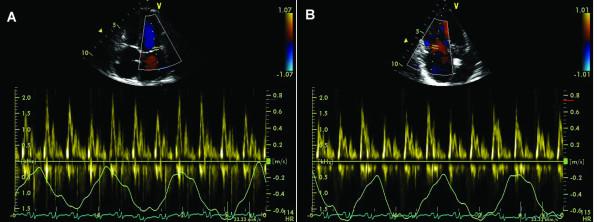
Constrictive pericarditis. A (left): respiratory variations of transmitral Doppler inflow: a decrease of peak flow velocity with > 25% (from 2.1 to 1.5 m/s) during inspiration. B (right): respiratory variations of tricuspid inflow (increased flow velocity during inspiration from 1.6 to 1.9 m/s).
